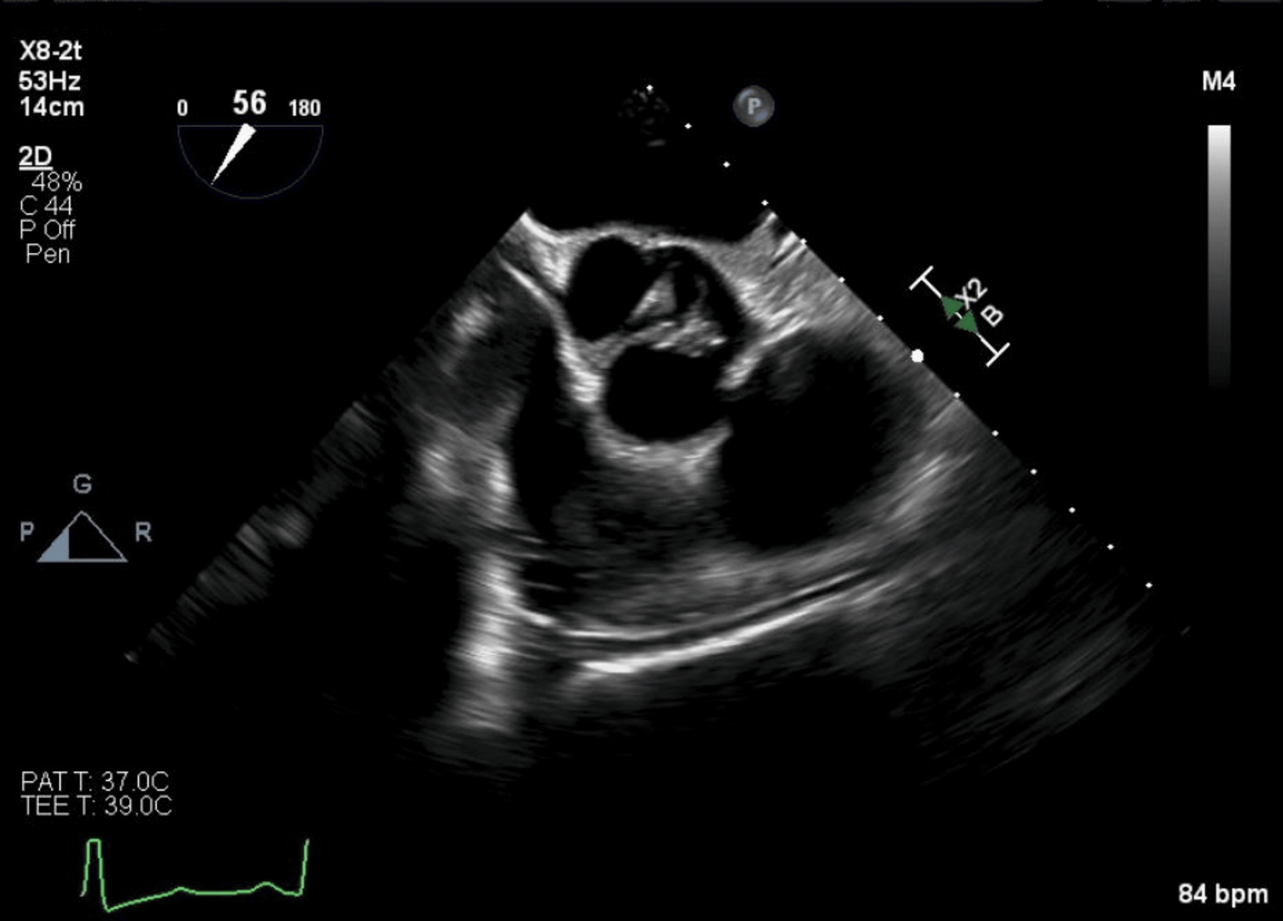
TEE aortic valve short axis view showing vegetation present at the commissure of the left and right coronary cusps TEE: transesophageal echocardiography
